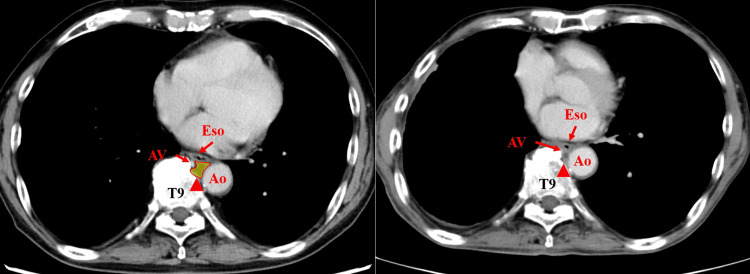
Comparison of CT findings before and after thoracic duct ligation. a. T9 levels (before lung cancer surgery): The red triangular arrow shows the adipose tissue containing the thoracic duct surrounded by the azygos vein, descending aorta, and esophagus. b. T9 level (after thoracic duct ligation): The red triangular arrow shows the postoperatively observed defect in the adipose tissue surrounded by the azygos vein, descending aorta, and esophagus. Ao, aorta; AV, azygos vein; Eso, esophagus; T, thoracic vertebra.
